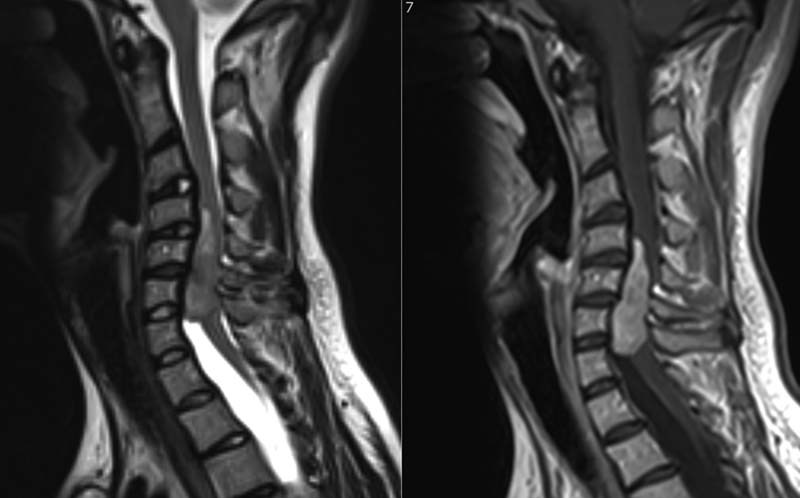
Sagittal (left) T2-weighted and (right) T1-weighted magnetic resonance imaging of the second case showing an intradural extramedullary tumor with intermediate and bright signal intensity, respectively, extending opposite fourth to seventh cervical vertebrae.
